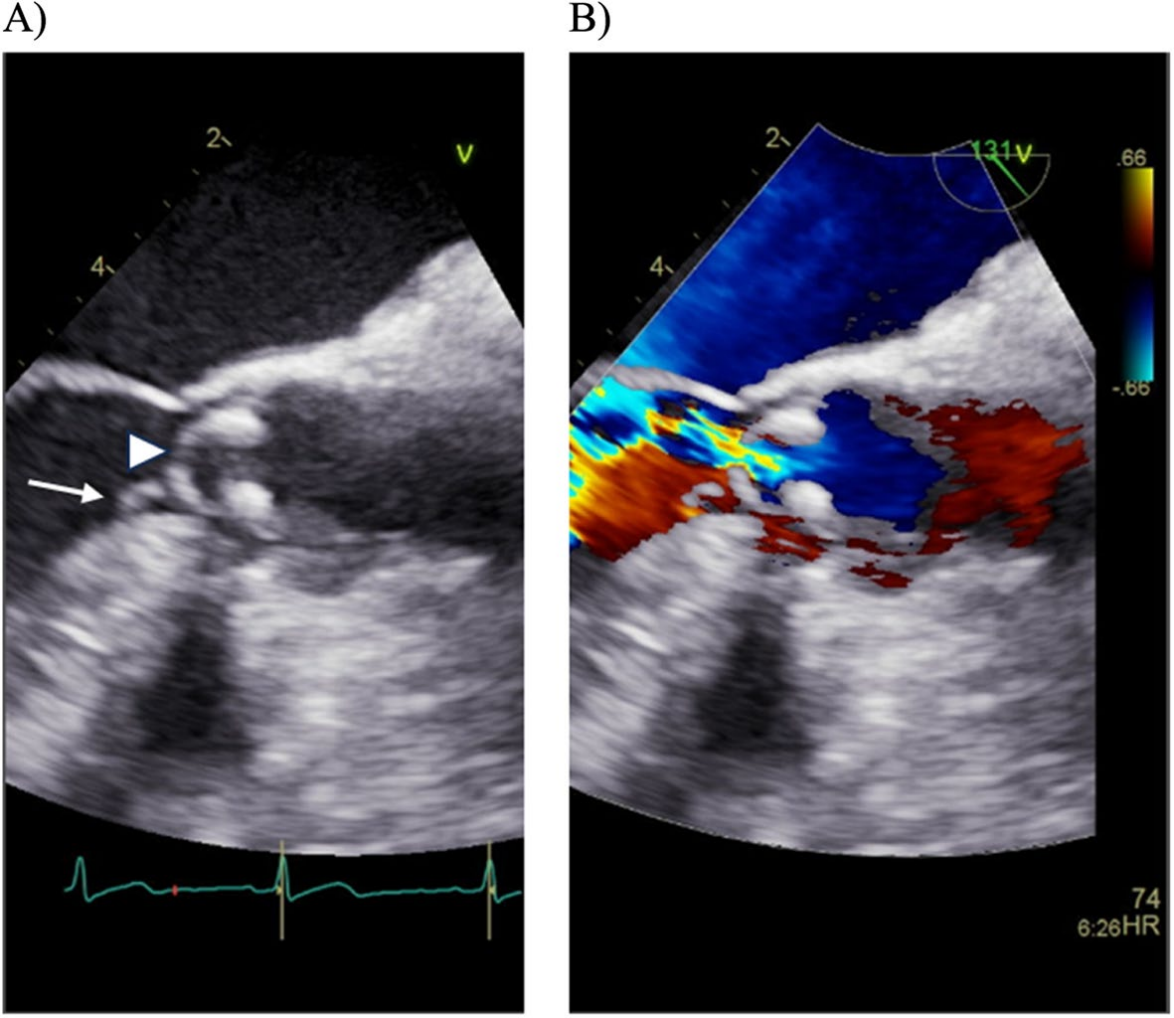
Images of transesophageal echocardiography (TEE). On the TEE, a vegetation (4.8 × 2.8 mm) on the noncoronary cusp (white arrow) and aneurysmal enlargement of noncoronary cusp with perforation (white arrow head) were identified (A). A severe aortic regurgitation from perforated noncoronary cusp was demonstrated by TEE with Color Doppler echocardiography (B)
